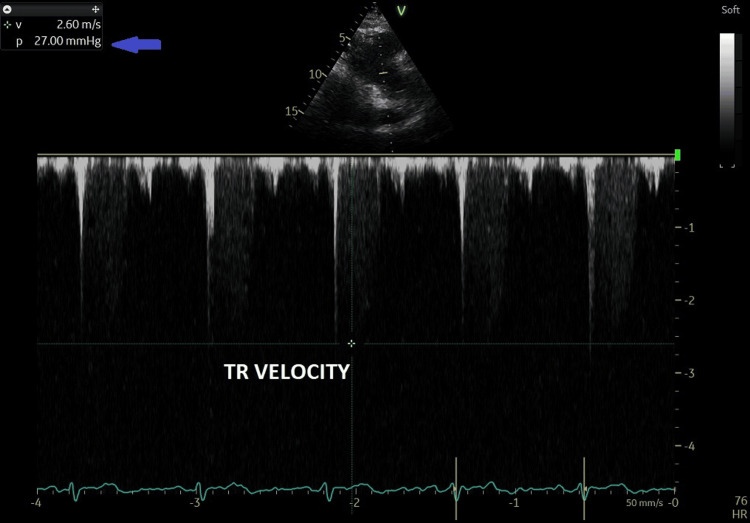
Tricuspid regurgitation velocity recording using continuous wave Doppler at 27 mmHg (blue arrow). TR VELOCITY = tricuspid regurgitation velocity; p = pressure gradient; V = velocity
